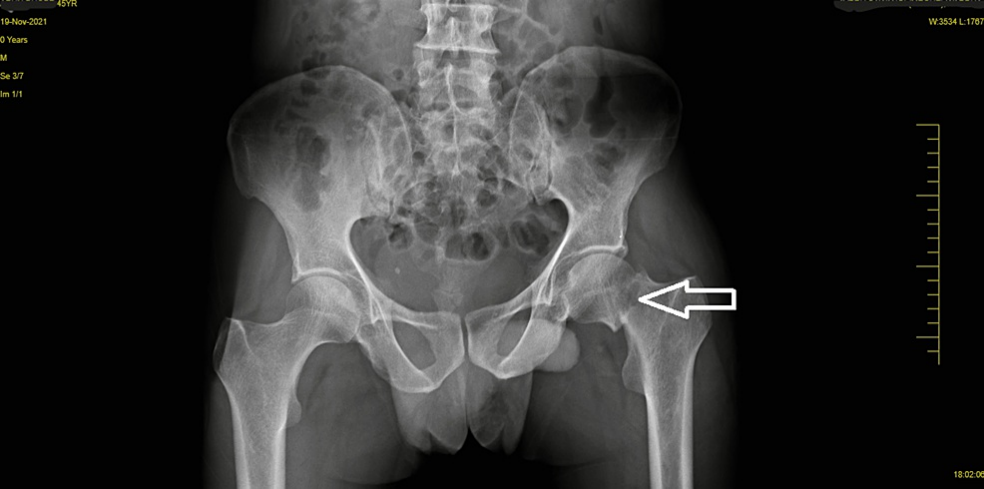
X-ray of the hip (AP view) shows a fracture of the femoral neck. AP view: anteroposterior.
